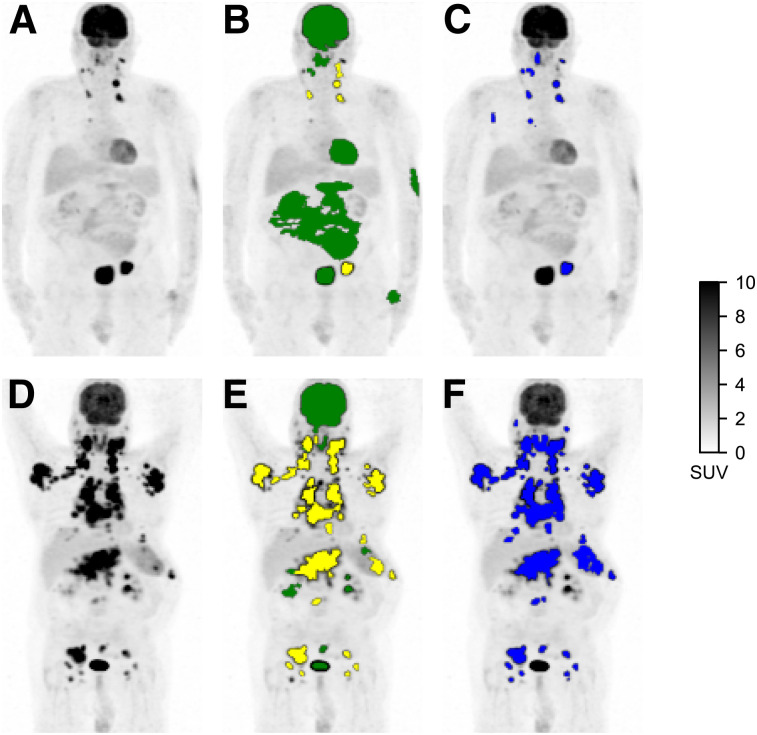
Detection of regions of high ^18^F-FDG uptake and classification as physiologic or suspicious. (A and D) Maximum-intensity-projection PET images of subjects with low TMTV (A) and high TMTV (D). (B and E) ROI_PARS_ obtained automatically using PARS software prototype. ROI_PARS_ sites detected by MFS algorithm are overlaid onto PET maximum-intensity projection. ROI_PARS_ sites classified by deep-learning algorithm as physiologic are shown in green, and ROI_PARS_ sites classified as suspicious are shown in yellow. (C and F) ROI_REF_ obtained by an experienced nuclear medicine physician using semiautomatic software.
